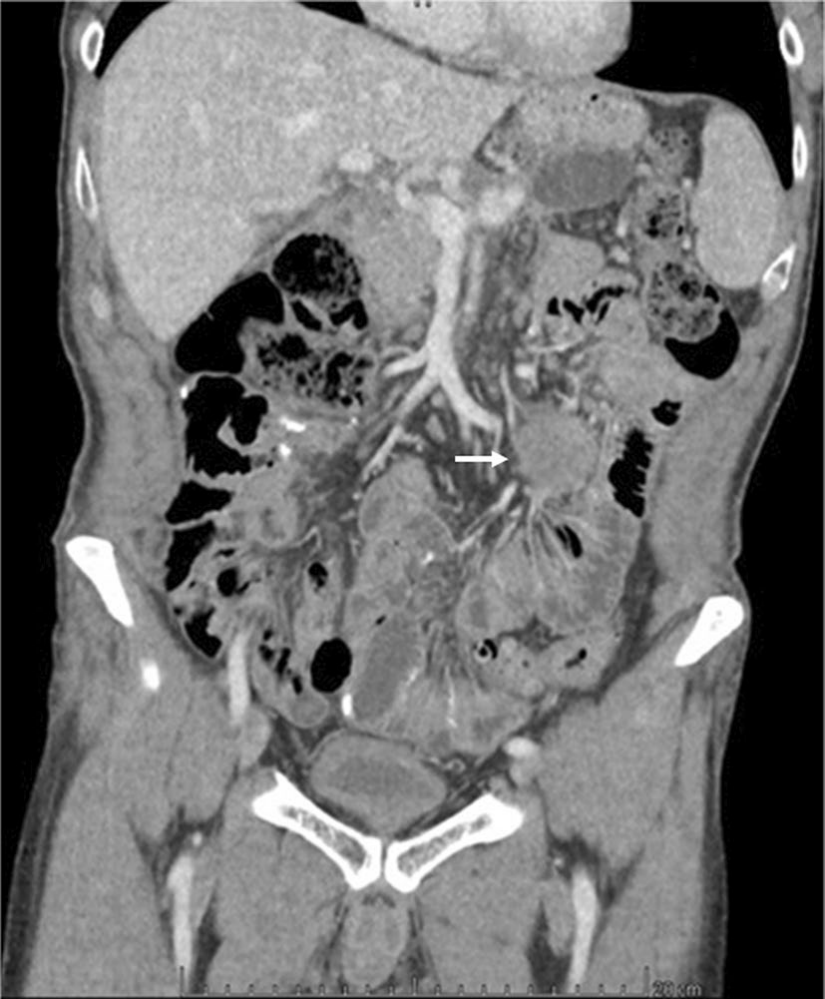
Coronal scan of contrast CT three months after resection of three intraabdominal sporadic desmoids: the patient was symptomatic for abdominal pain; the fourth lesion, located at the mesentery of the first jejunal loop, had increased in volume with respect to previous control, and there were signs of vascular and bowel compression
